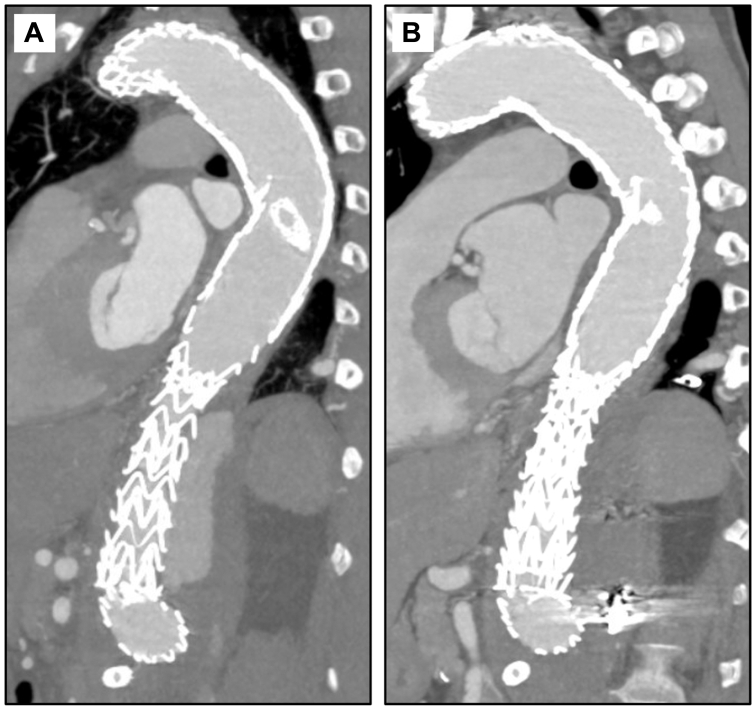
Case 2 computed tomography angiograms. (A) Preoperative sagittal view shows extensive endoleak into the false lumen (FL). (B) One-month postoperative sagittal view showing interval resolution of endoleak with some sac remodeling around Shape memory plugs (SMPs) and candy plug within the thrombosed FL.
